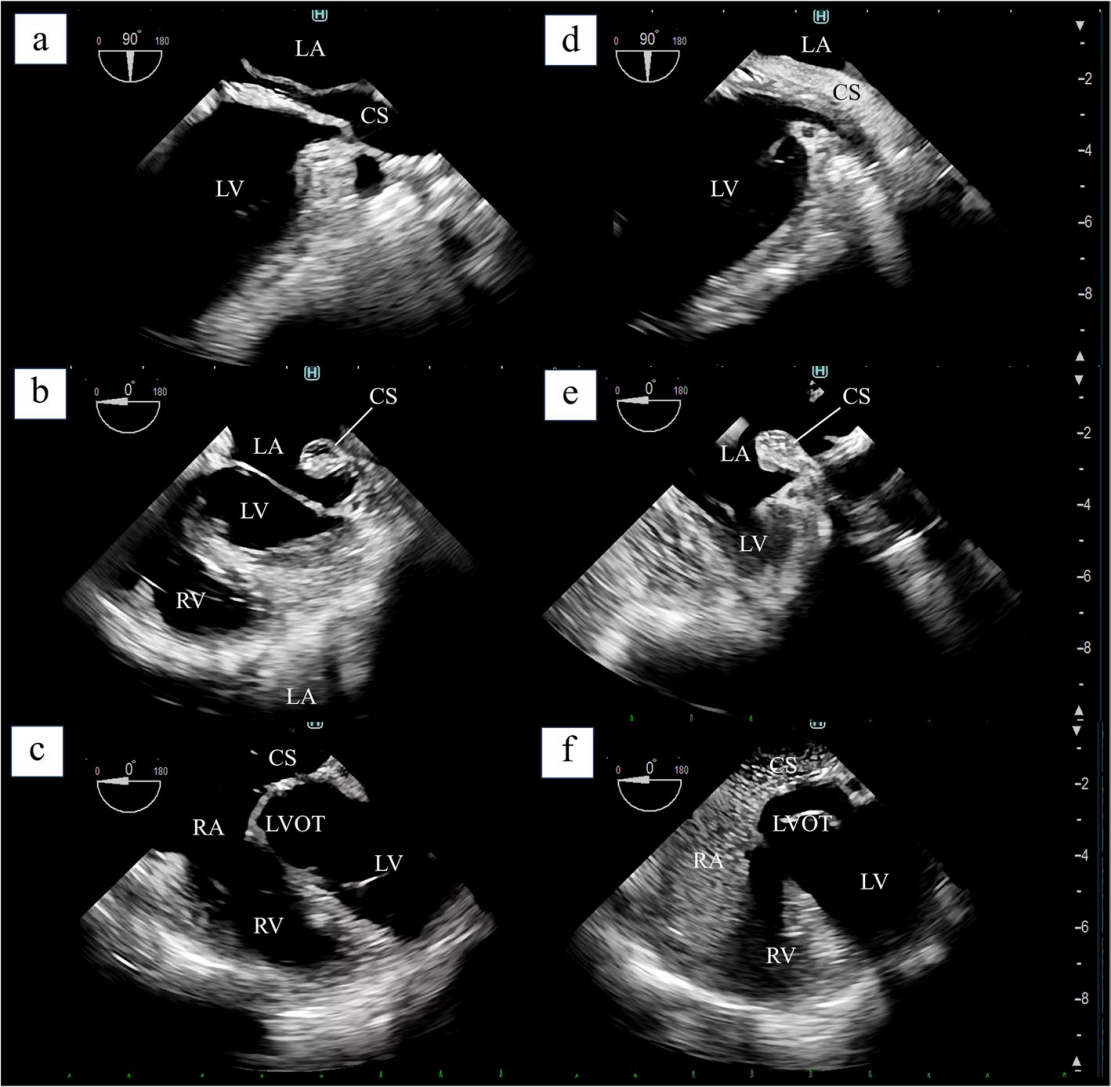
Intraoperative transesophageal echocardiography and agitated saline test after unroofed coronary sinus repair. a Short-axis view and b long-axis view of URCS after direct fistula closure. c Close to the five-chamber view with an enlarged CS opening to the right atrium. d, e, f The agitated saline test was performed again after URCS repair from the left upper extremity, which easily and reliably confirmed that there was no residual leakage from the CS into the left ventricular system. CS, coronary sinus; LA, left atrium; LV, left ventricle; RA, right atrium; RV, right ventricle; LVOT, left ventricular outflow tract
